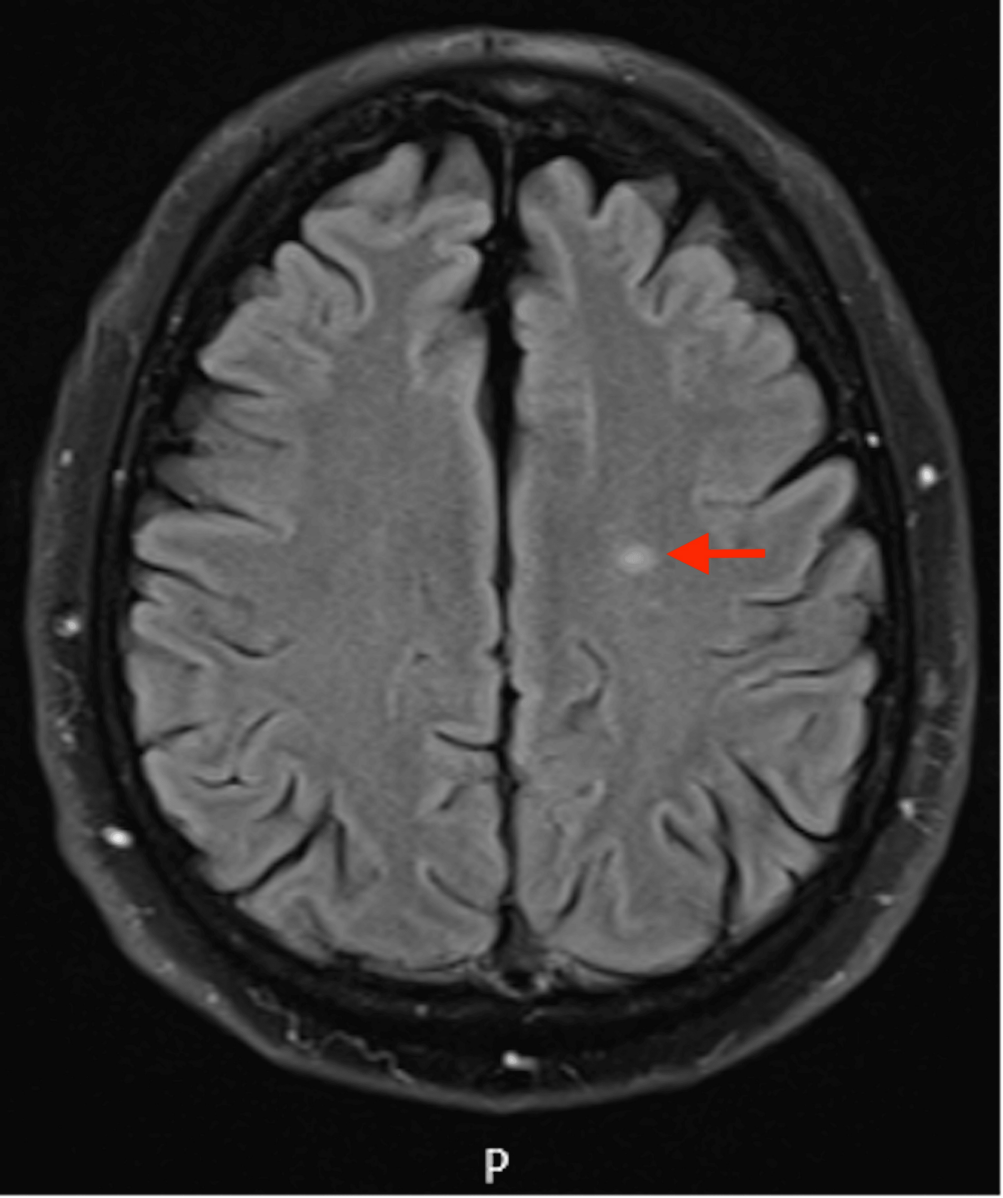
Axial FLAIR MRI showing a small infarct in the left centrum semiovale FLAIR: Fluid-attenuated inversion recovery
